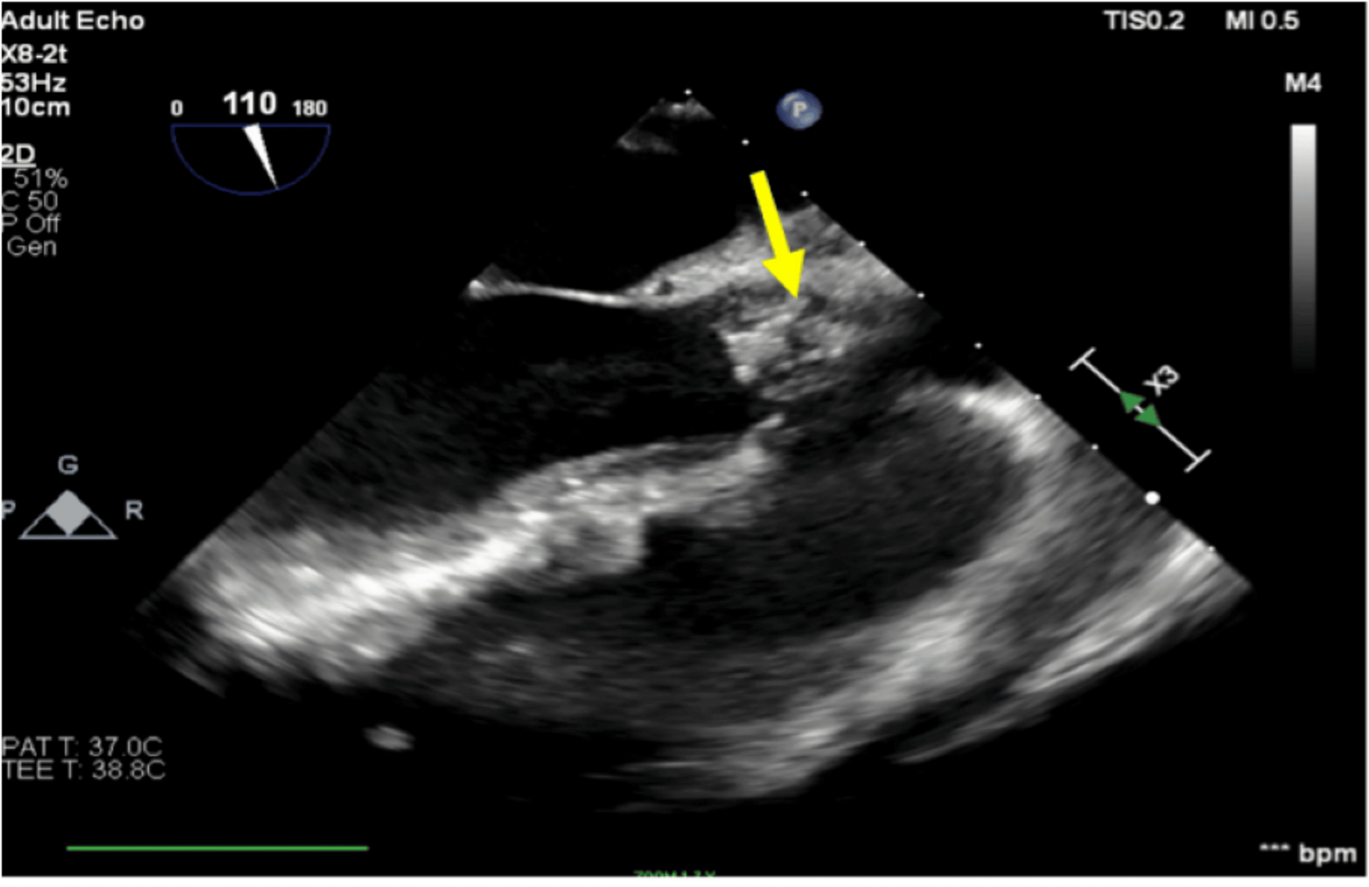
Transesophageal echocardiogram (TEE) findings TEE showing a long-axis view of the aortic valve and proximal aorta. The arrow shows a large conglomerate mass encasing the right and non-coronary cusps prolapsing towards the coronary ostia in systole.
